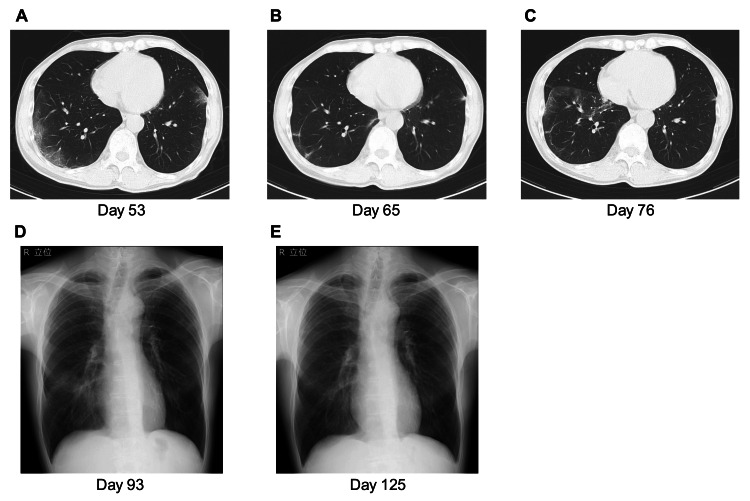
Chest computed tomography (CT) and X-ray images (A) Chest CT on day 53, in the first relapse, showing multiple bilateral ground-glass opacities (GGOs). (B) Chest CT on day 65, the first remission after five days of remdesivir, showing the resolution of most of the GGOs. (C) Chest CT on day 76, in the second relapse, showing GGO recurrence. (D) Chest X-ray on day 93, the third relapse, showing opacities in the right lower lung. (E) Chest X-ray on day 125, after 30 days of nirmatrelvir/ritonavir, showing resolution of the opacities.
